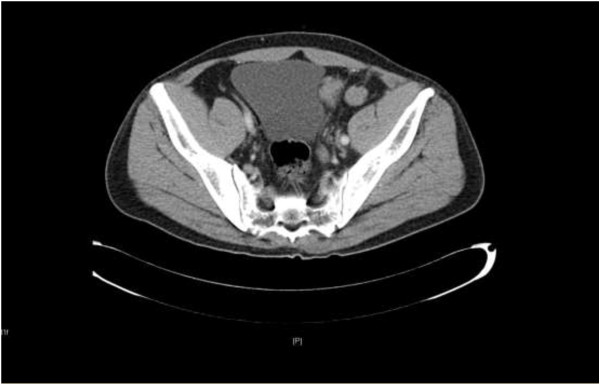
Computed tomography showed multiple lesions in left inguinal region, and near-bilateral iliac vessels, suggestive of multiple metastases.
